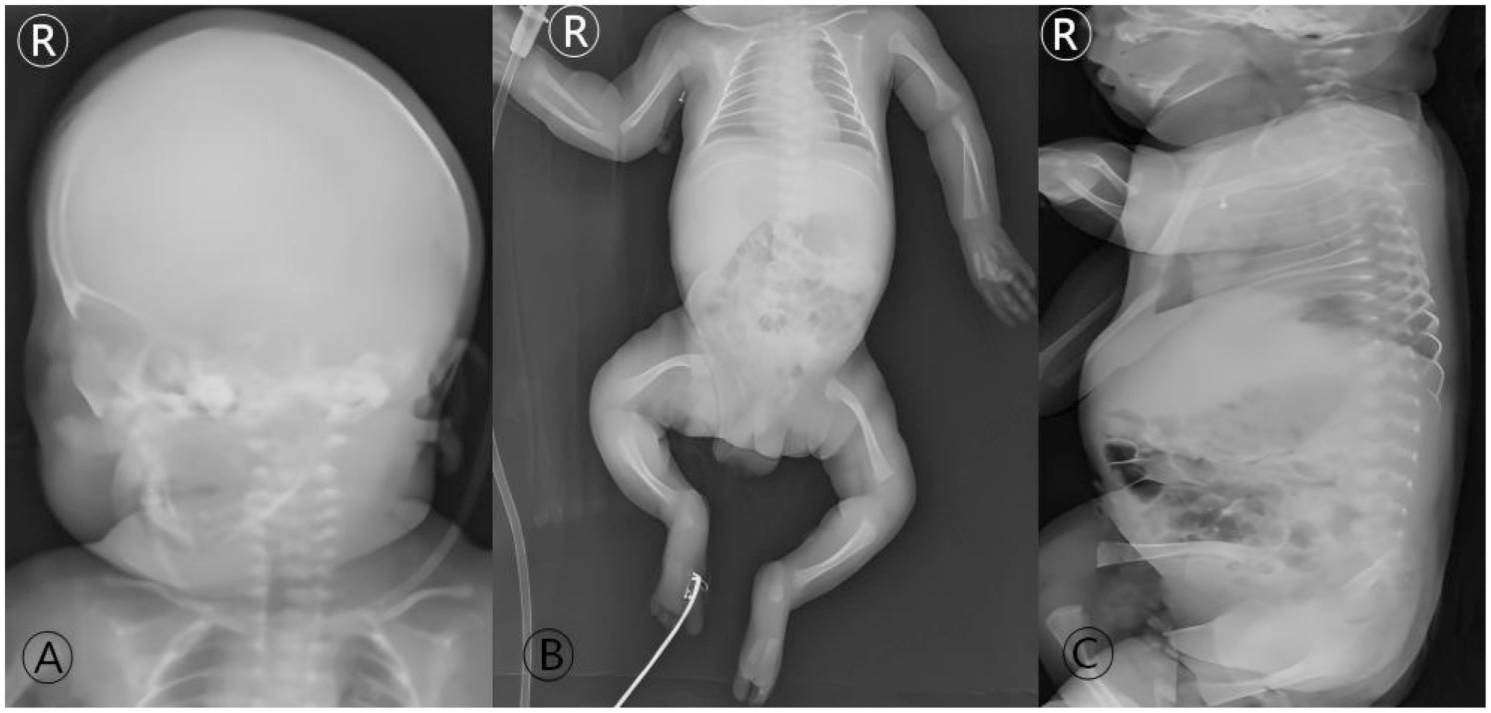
Initial radiographic skeletal survey at admission (Day 9 of life). Full-body radiographs demonstrate the classic imaging features of severe, type III OI: (A) diffuse osteopenia and thinning of the cranial vault. (B) Generalized osteopenia of the long bones, thin cortices, relatively widened medullary cavities, and flared metaphyses; slender and curved bilateral clavicles are also evident. (C) Loss of normal spinal curvatures. These findings form the radiographic basis for the diagnosis of OI in the neonatal period and explain the inherent high fracture risk.
